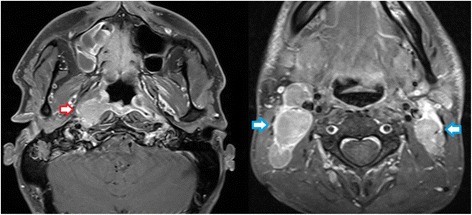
T1-weighted MRI in November of 2010 showing contrast-enhancing right-sided nasopharyngeal primary tumor (red arrow) as well as bilateral metastatic lymph nodes of the neck (blue arrows)
